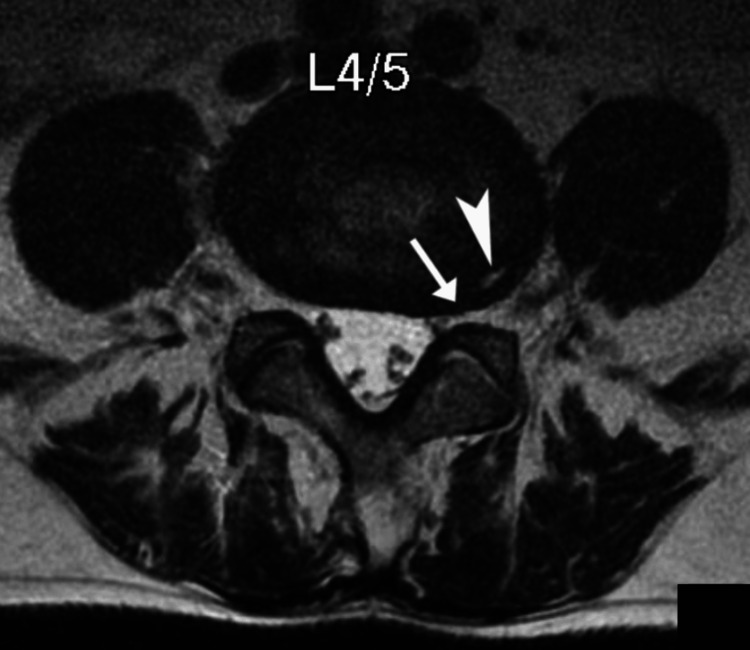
T2-weighted axial lumbar magnetic resonance image at the level of the L4/5 intervertebral disc A left-sided posterior annular fissure (arrowhead) is evident, while a disc bulge impresses the thecal sac and causes mild left neural foraminal stenosis (arrow).
